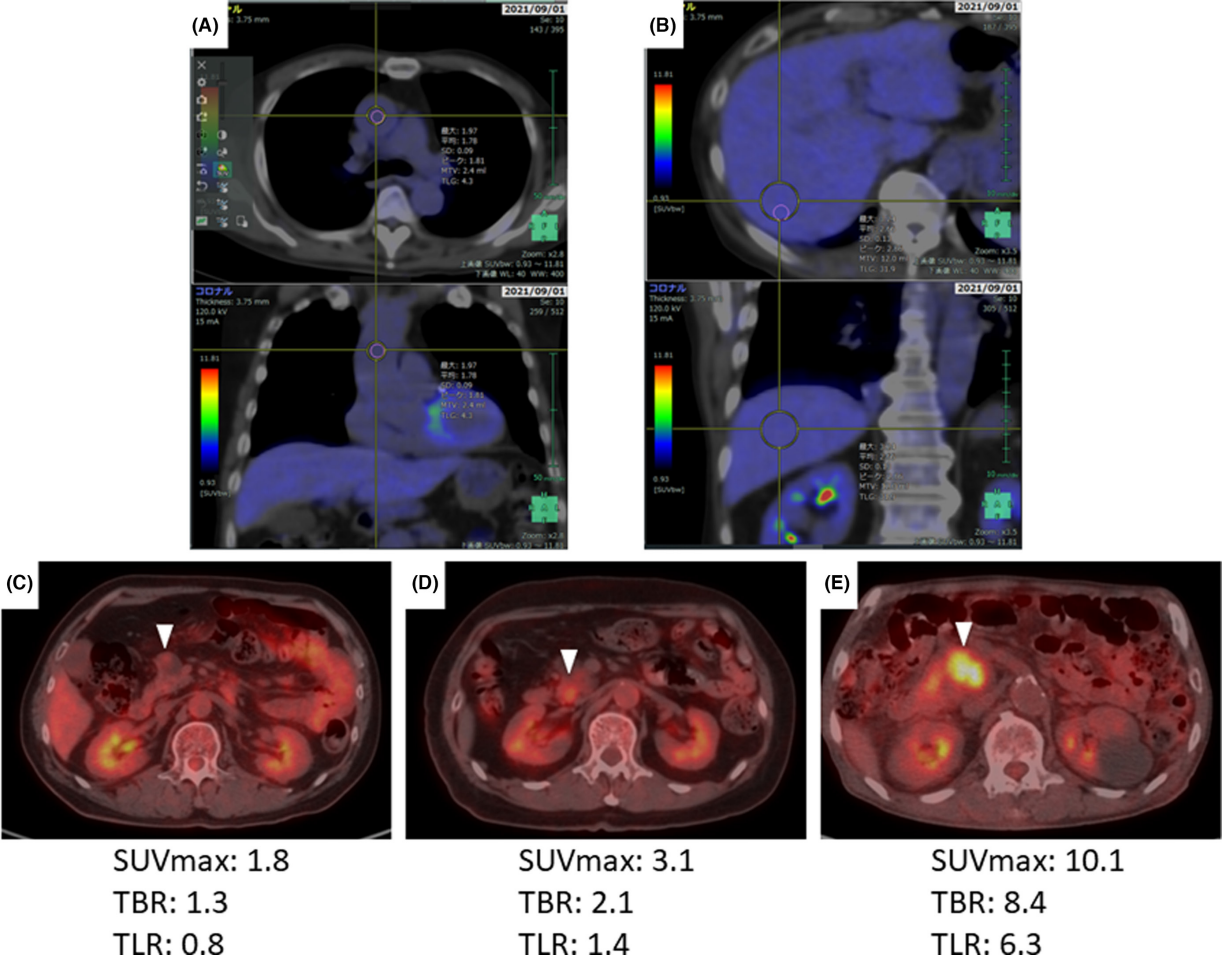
Measurements of SUV in FDG‐PET/CT. A, SUV mean measurements in the blood pool of the ascending aorta. B, SUV mean measurement in the right lobe of the liver. C, Typical example of PET‐CT image for IPMA. The white arrow indicates the site of accumulation. D, Typical example of PET‐CT image for HGD. The white arrow indicates the site of accumulation. After formalin fixation, HGD portion was widely distributed within the tumor (maximum diameter: 23 mm). The FDG‐accumulated area (maximum diameter: 14.5 mm) generally corresponded to the distribution of the HGD portion. E, Typical example of PET‐CT image for IPMC. The white arrow indicates the site of accumulation
